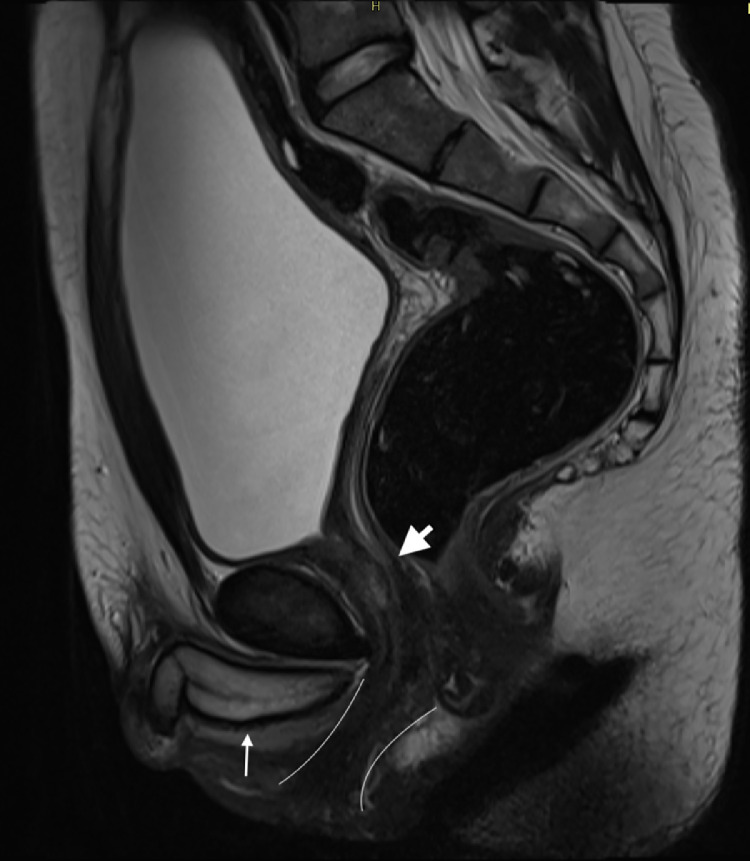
Sagittal T2-weighted MR image shows a hypertrophied clitoris with corpora cavernosa forming a penis (thin arrow), periurethral prostatic tissue (arrowhead), and a common urogenital sinus (outlined by curved lines).
